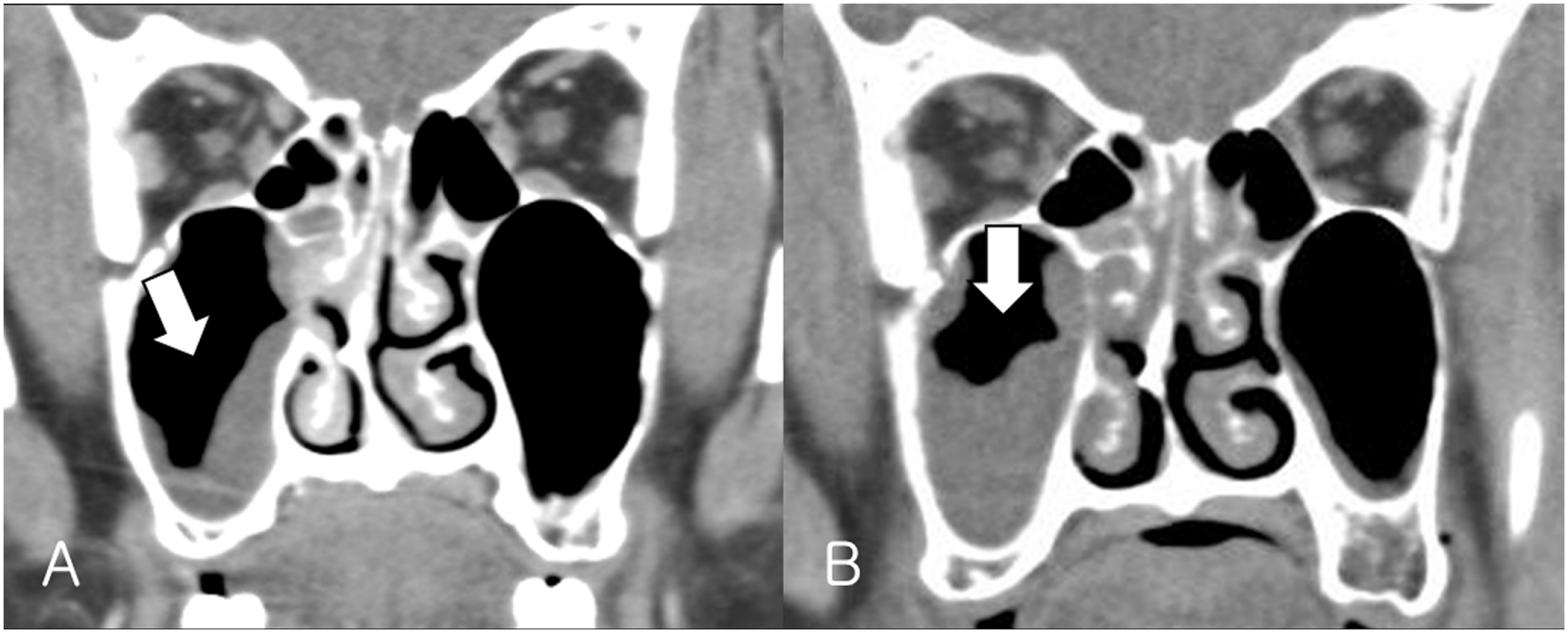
Computed tomography (CT) scans of a patient with rhino-orbito-cerebral mucormycosis and mucosal thickening. A. Initial CT scan of a patient who presented with complaints of eyelid swelling and orbital pain. Coronal view shows mucosal thickening in the right maxillary sinus and opacification in some of the ethmoid air cells; B. Follow-up CT scan 5 days after the first visit. Mucosal thickening in the right maxillary sinus had progressed.
